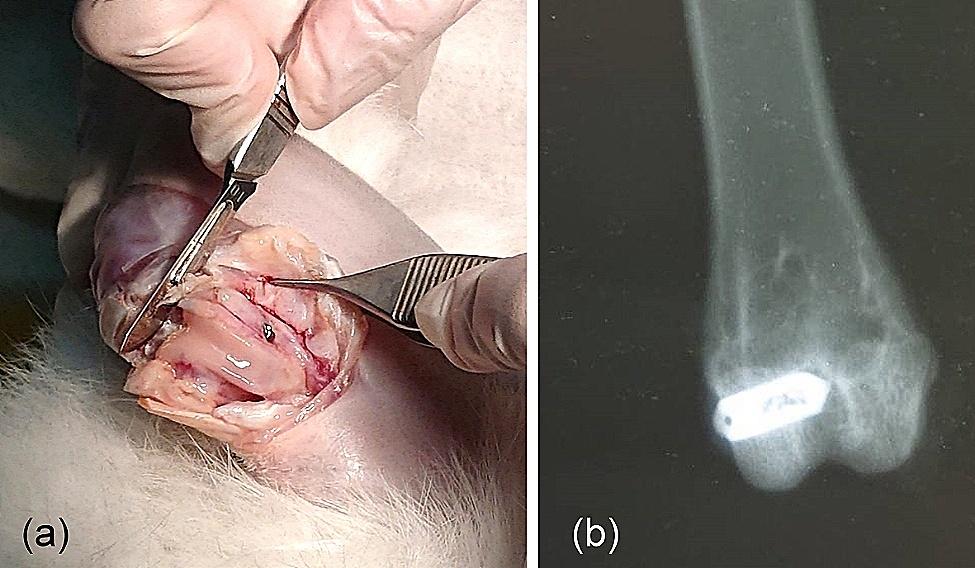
(a) Image to demonstrate the exposure of the lateral femoral condyle, location of the drilled hole and SA inserted along the axis of the hole. (b) The lateral femoral condyle was the site where the SA was inserted, as indicated by the radiograph
